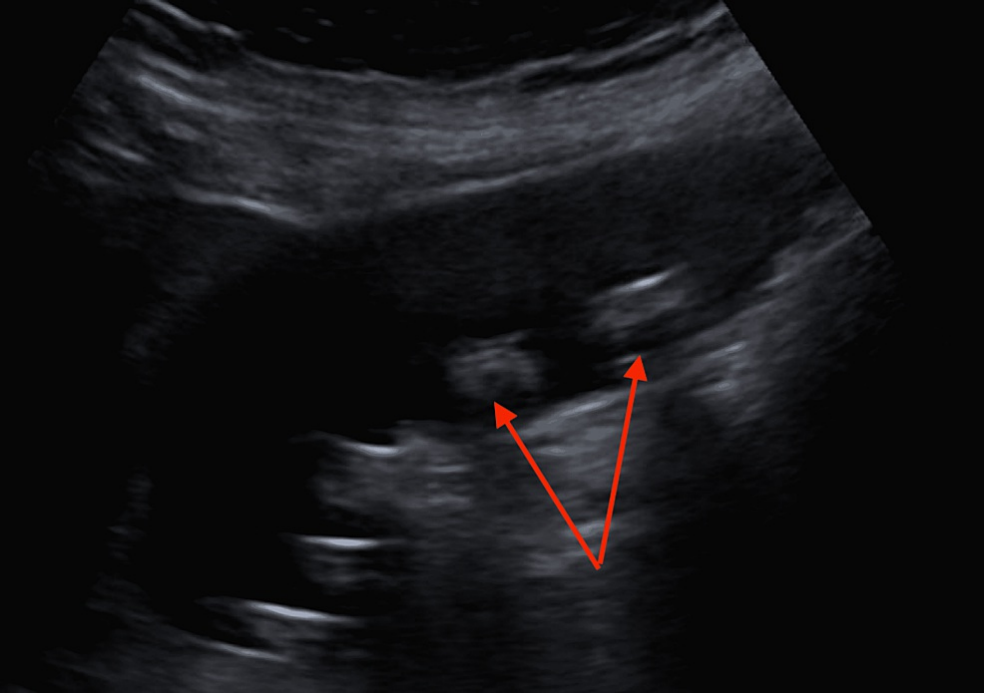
Right upper quadrant ultrasound Red arrows pointing to gallstones within the gallbladder
